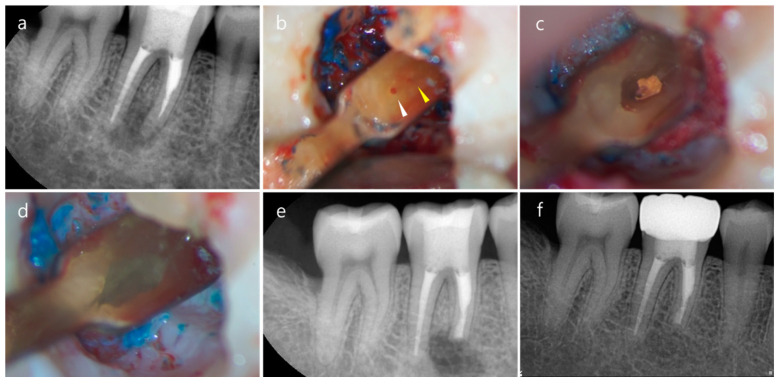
Endodontic microsurgery on an isthmus-absent mandibular molar. (a) A preoperative periapical radiograph shows periapical radiolucency and unsatisfactory canal filling on the mesial root due to an unnegotiable ledge. (b) The resected root surface shows mesio-buccal (white triangle) and mesio-lingual (yellow triangle) canals, which are closely located without an isthmus at 3 mm from the apex. (c) The simple, oval-shaped root-end cavity. (d) The root-end filling with mineral trioxide aggregate (MTA). (e) A postoperative periapical radiograph. (f) A 15-month follow-up radiograph shows that complete healing occurred.
